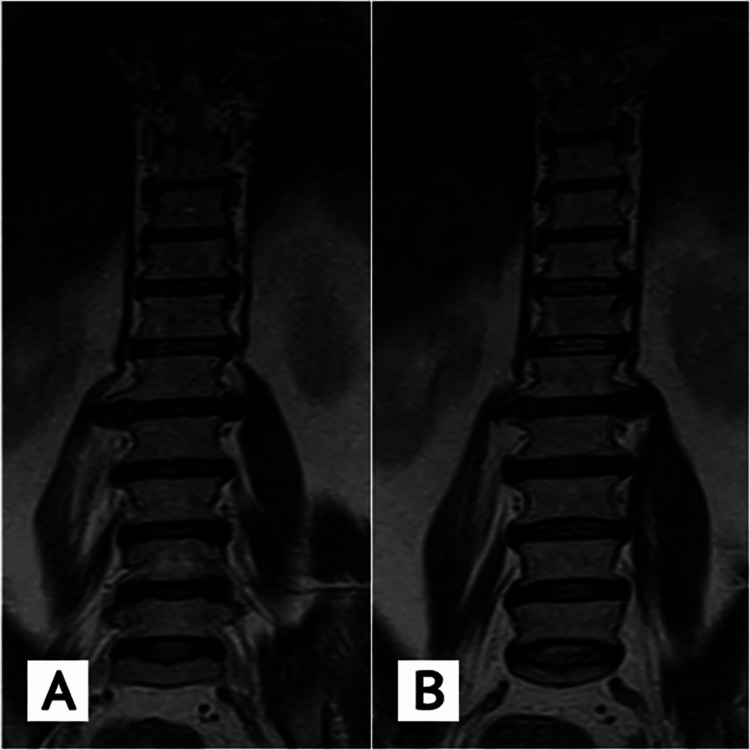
T2 coronal MRI images of the lumbosacral spine show (A & B) a large lateral disc osteophyte complex more on the right side indenting the right psoas muscle with an abnormal bright signal noted along the whole muscle.
